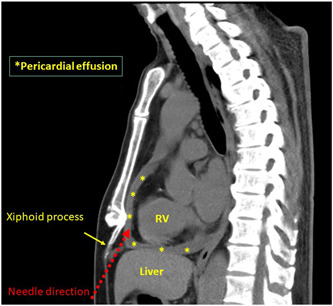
Reconstruction of the sagittal axis view of the chest CT. The Xiphoid process could be a suitable landmark for the subxiphoid anterior approach. The red dotted arrow indicates the needle direction into the pericardial space with a subxiphoid approach. The asterisks indicate the distribution of the pericardial effusion. CT, computed tomography; RV, right ventricle
